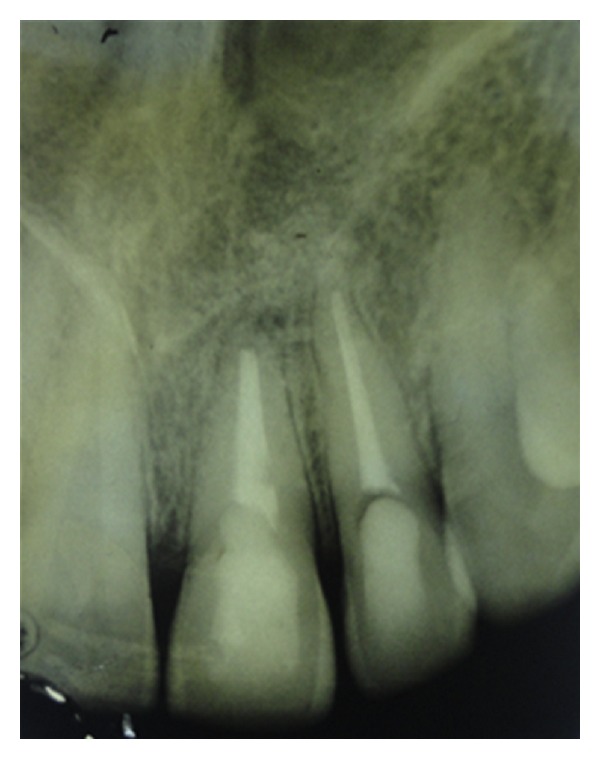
Follow-up radiograph after 9 months.
